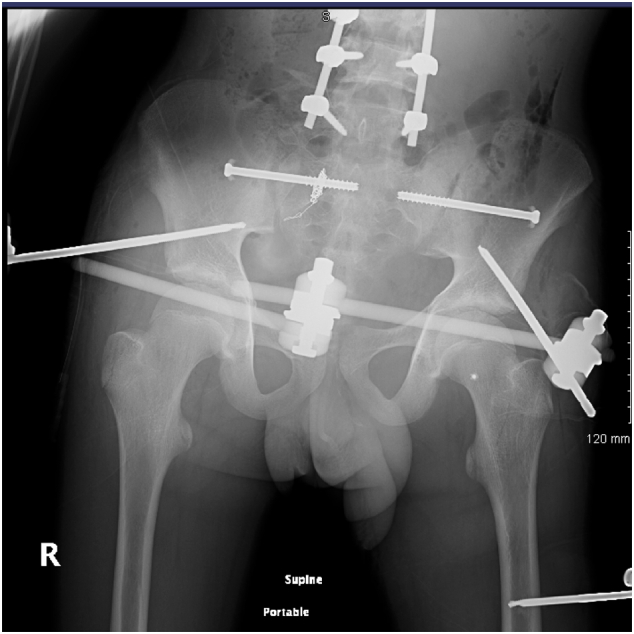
post operative radiograph with external fixator and two sacoilliac screws in situ and clips for the posterior branch of the right internal iliac artery.
